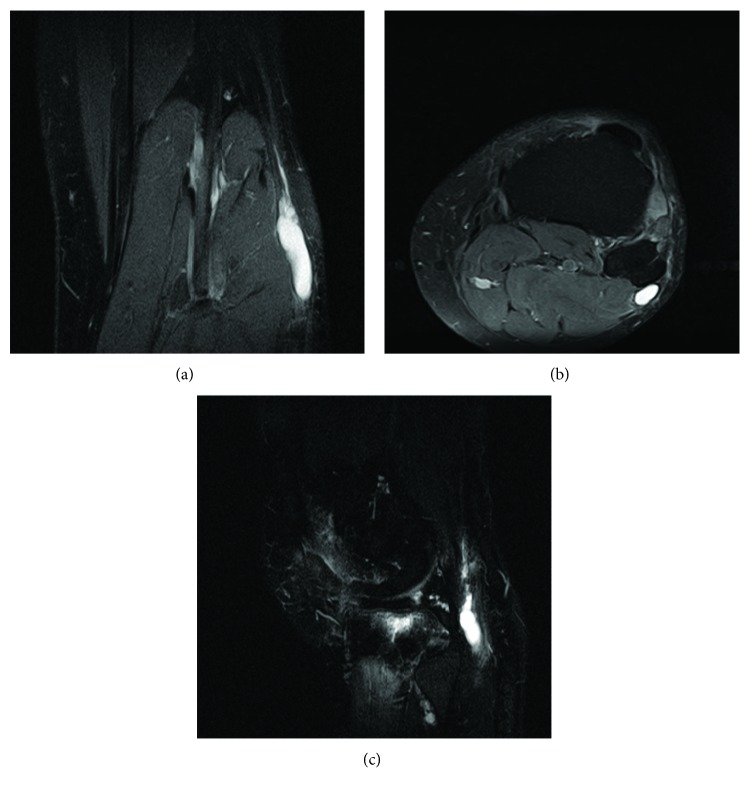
3 MRI views (sagittal, coronal, and axial) of the knee revealing a hyperintense fluid collection within the CPN with connection to the proximal tibiofibular joint, consistent with an intraneural ganglion cyst.
